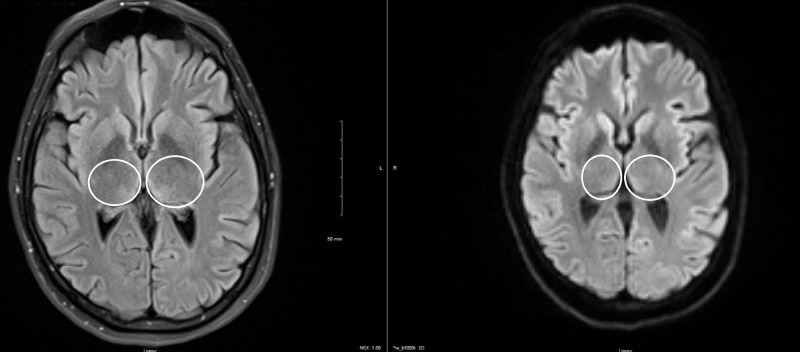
Follow-up images at three months Axial view of T2/FLAIR sequences shows the interval resolution of the previously seen hyperintense signal in bilateral thalamic regions (white circles) Abbreviations: FLAIR = fluid attenuation inversion recovery
